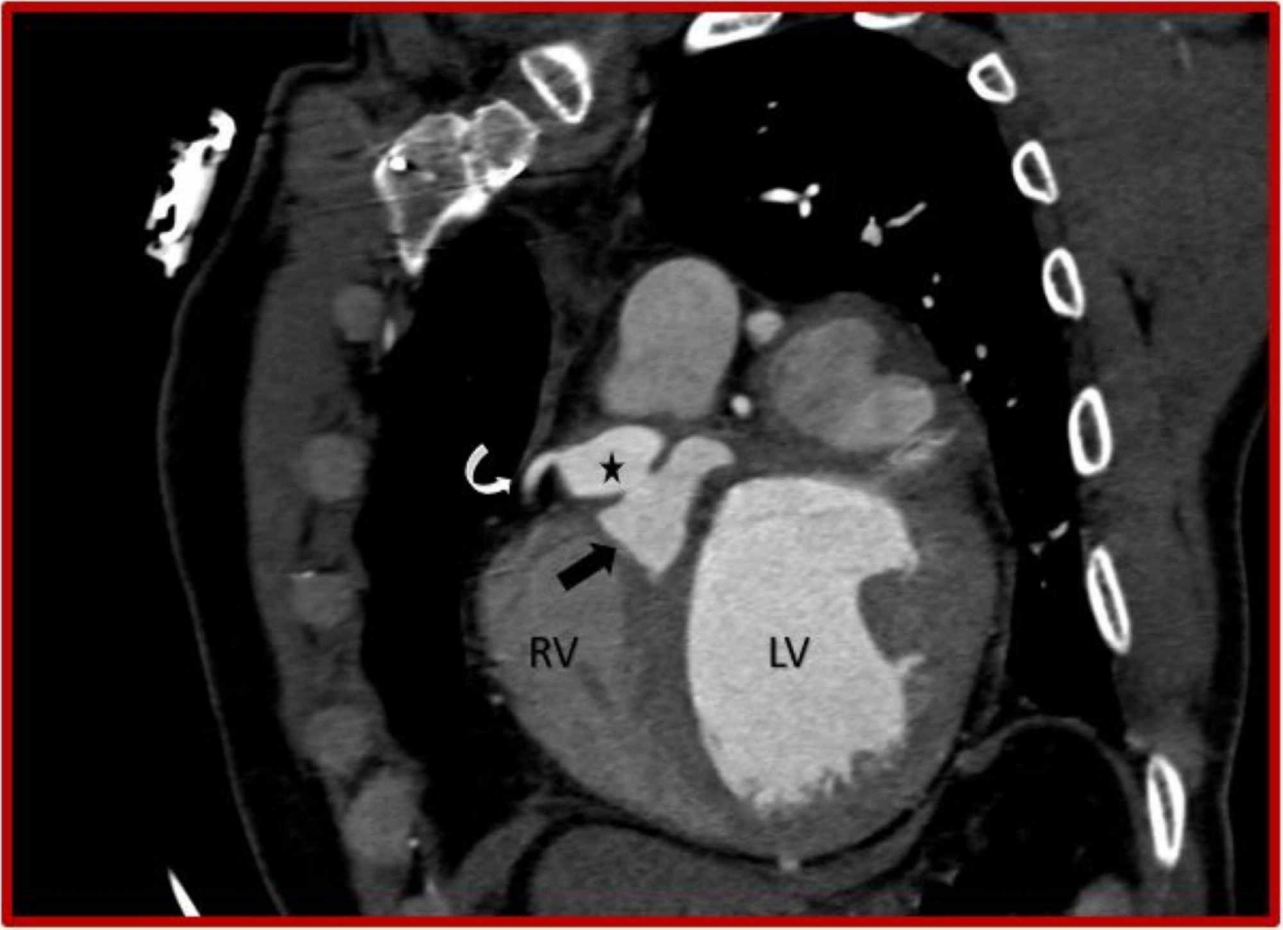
Cardiac CT (coronal oblique view) demonstrates ruptured right sinus of Valsalva aneurysm (asterisk) dissecting into the interventricular septum (black arrow) and right coronary artery (curved arrow) originating from the aneurysm. CT: Computed tomography; RV: Right ventricle; LV: Left ventricle.
